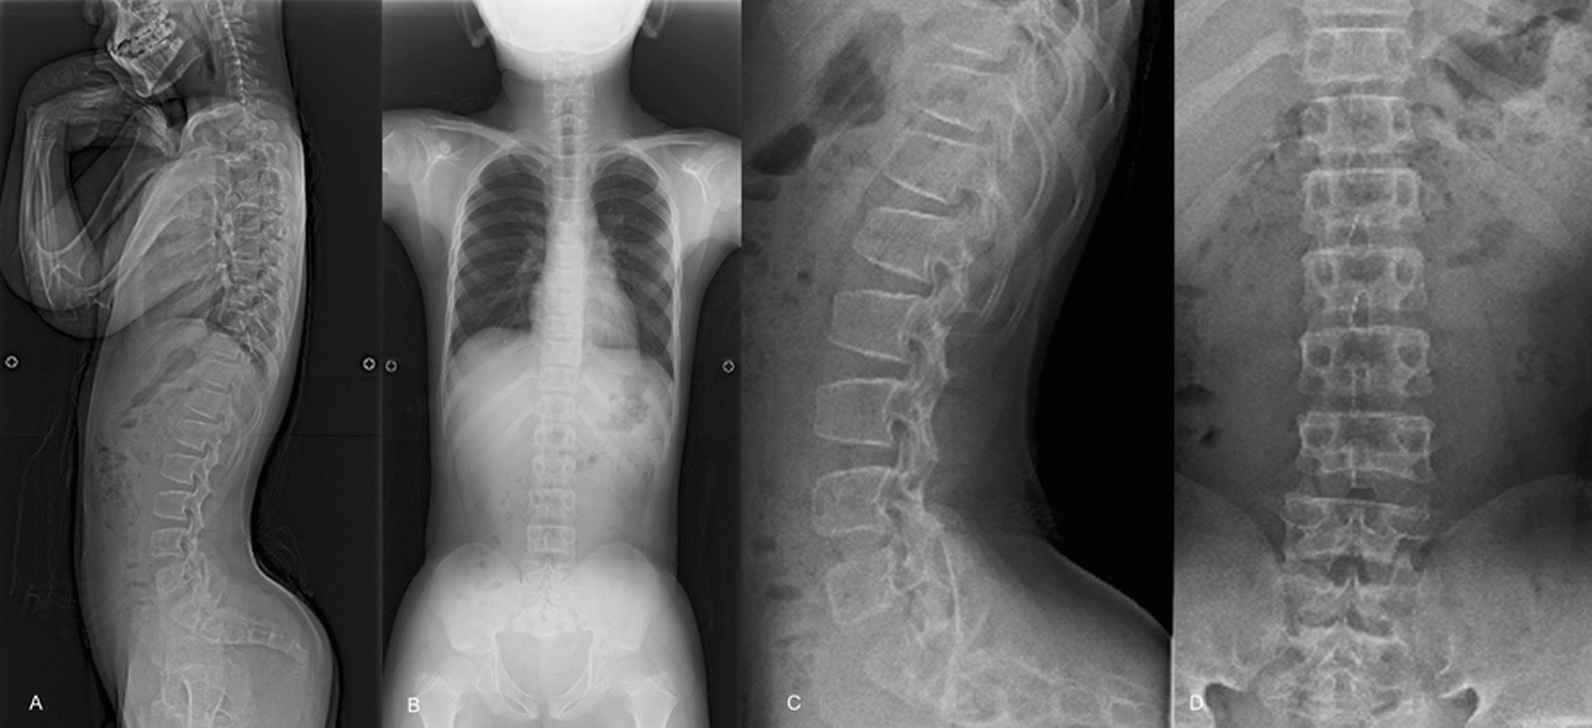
Preoperative whole-spine and lumbar X-ray images (A: lateral view of the whole spine, B: frontal view of the whole spine, C: lateral view of the lumbar spine, D: frontal view of the lumbar spine). These images revealed characteristic features of achondroplasia, such as hyperlordosis (L1–S1), a large pelvic tilt angle, and shortened pedicles. However, there was no significant evidence of thoracolumbar kyphosis
